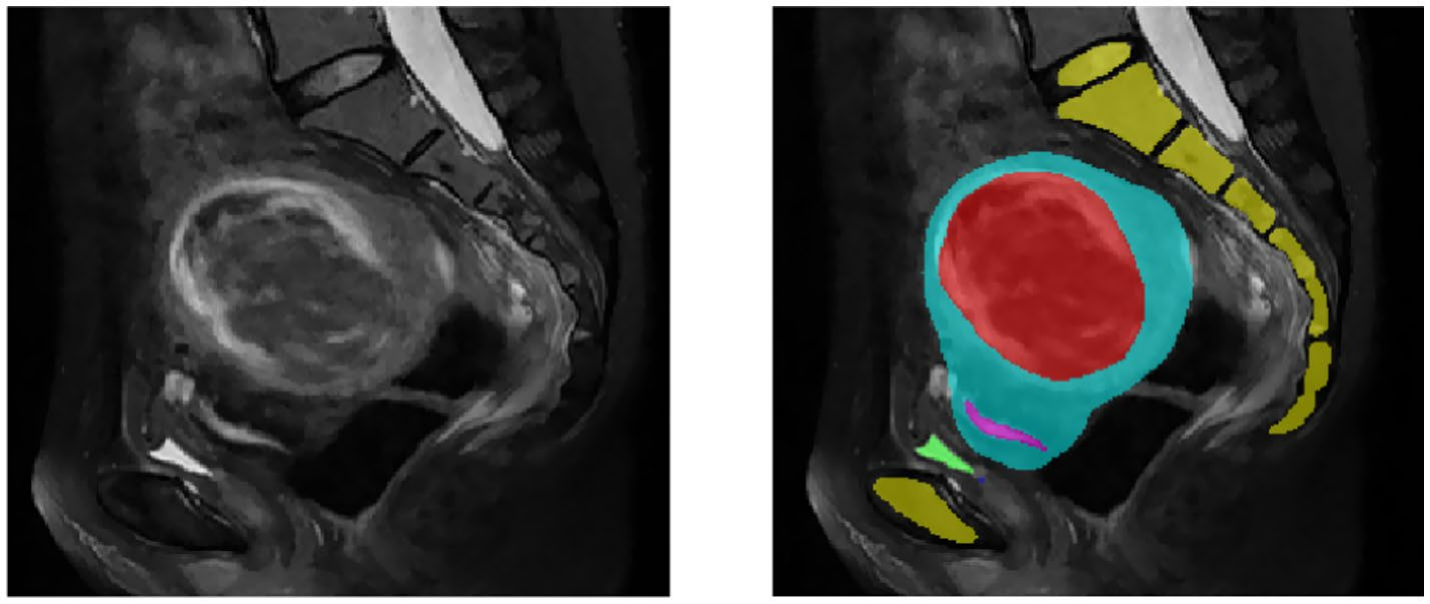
MR images of uterine fibroids. The yellow area is the spine, the red area is the fibroid, the blue area is the uterus, the pink area is the endometrium, and the green area is the bladder.
